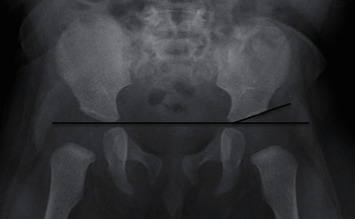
Normal anteroposterior radiograph of hips in 6-month-old boy shows that acetabular angles in the right and left hip (lines) are normal for age, measuring 22° and 24°, respectively (Starr et al. [25]).
